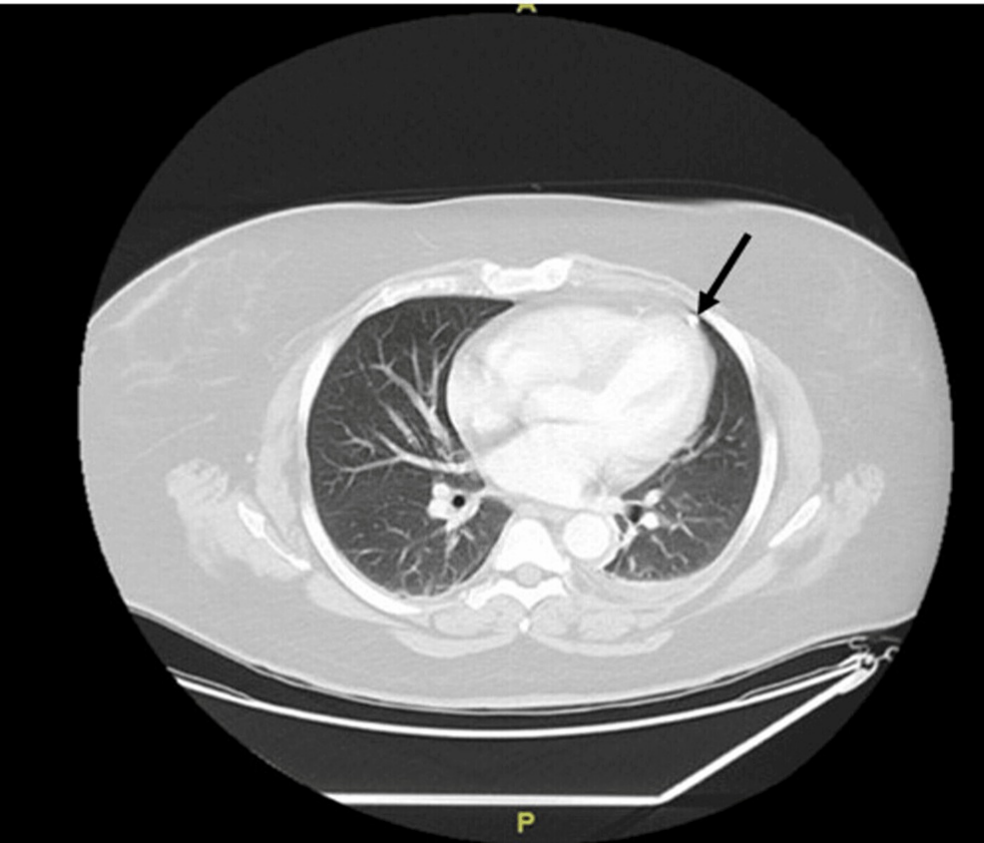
CT of chest with intravenous contrast showing mild dependent airspace disease with evidence of a calcified granuloma in left upper lung.
